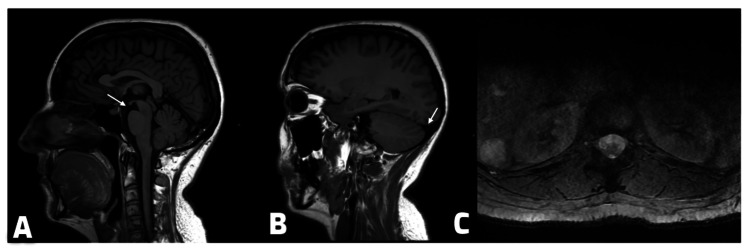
Follow-up imaging approximately 10 weeks after the first MRI. Sagittal T1 FLAIR brain images showing reduced brainstem sagging with an increased mamillopontine distance (6.5 mm) (A) and reduction in venous sinus engorgement (B). Axial 2D MERGE spine sequence demonstrating resolution of the anterior epidural collection (C). FLAIR: Fluid-attenuated inversion recovery; MERGE: Multi-Echo Recombined Gradient Echo.
